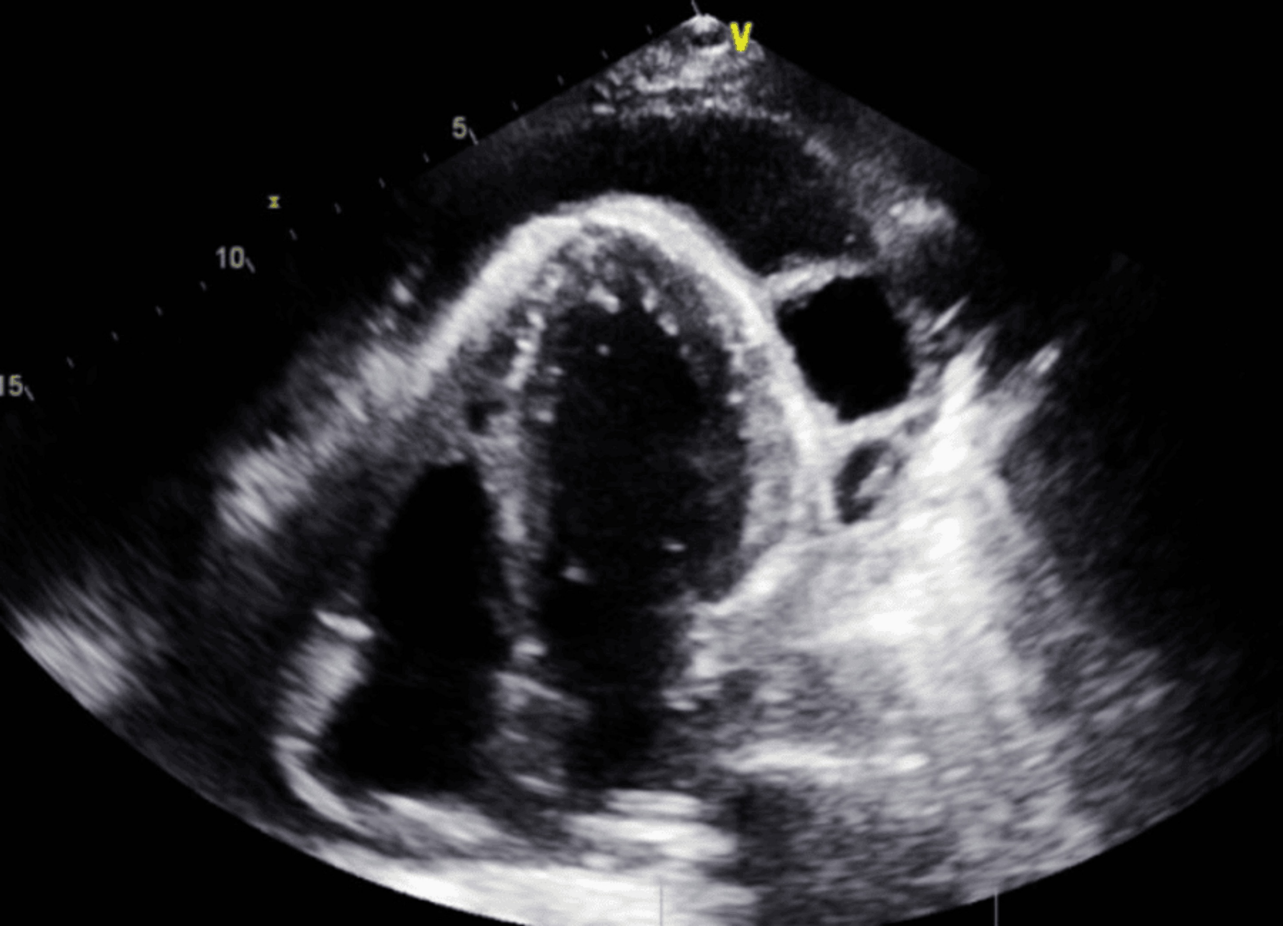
Echocardiogram on admission showing pericardial effusion
